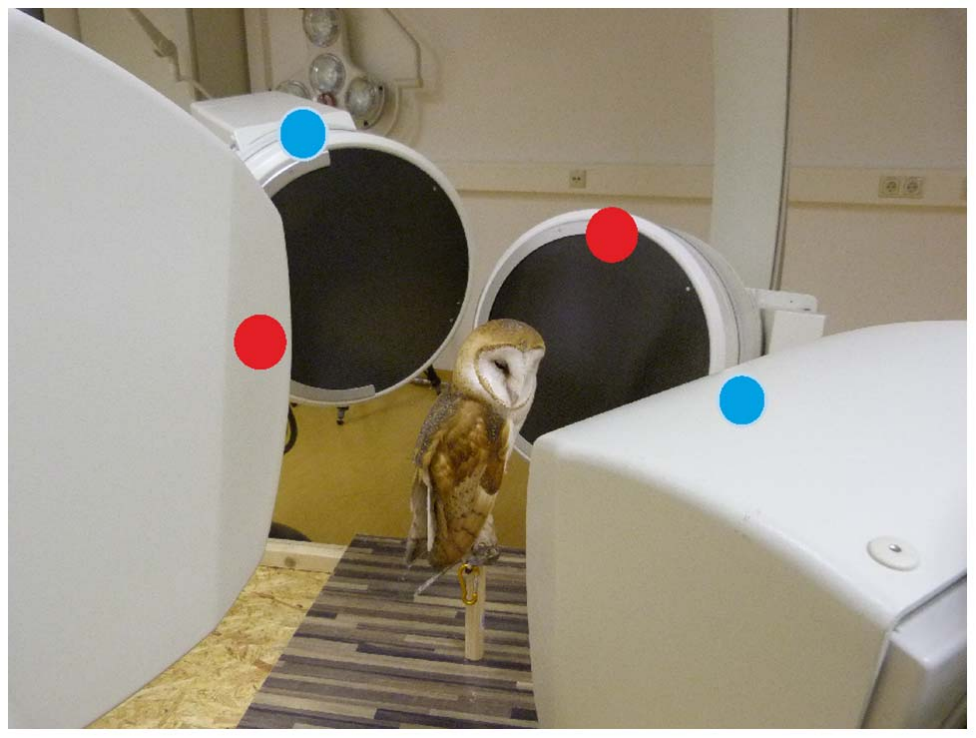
Photograph of an owl in the setup in Jena. The owl (*Tyto furcata pratincola*) is sitting on a perch with the head in the crossing point of the two X-ray systems (red and blue dots).
